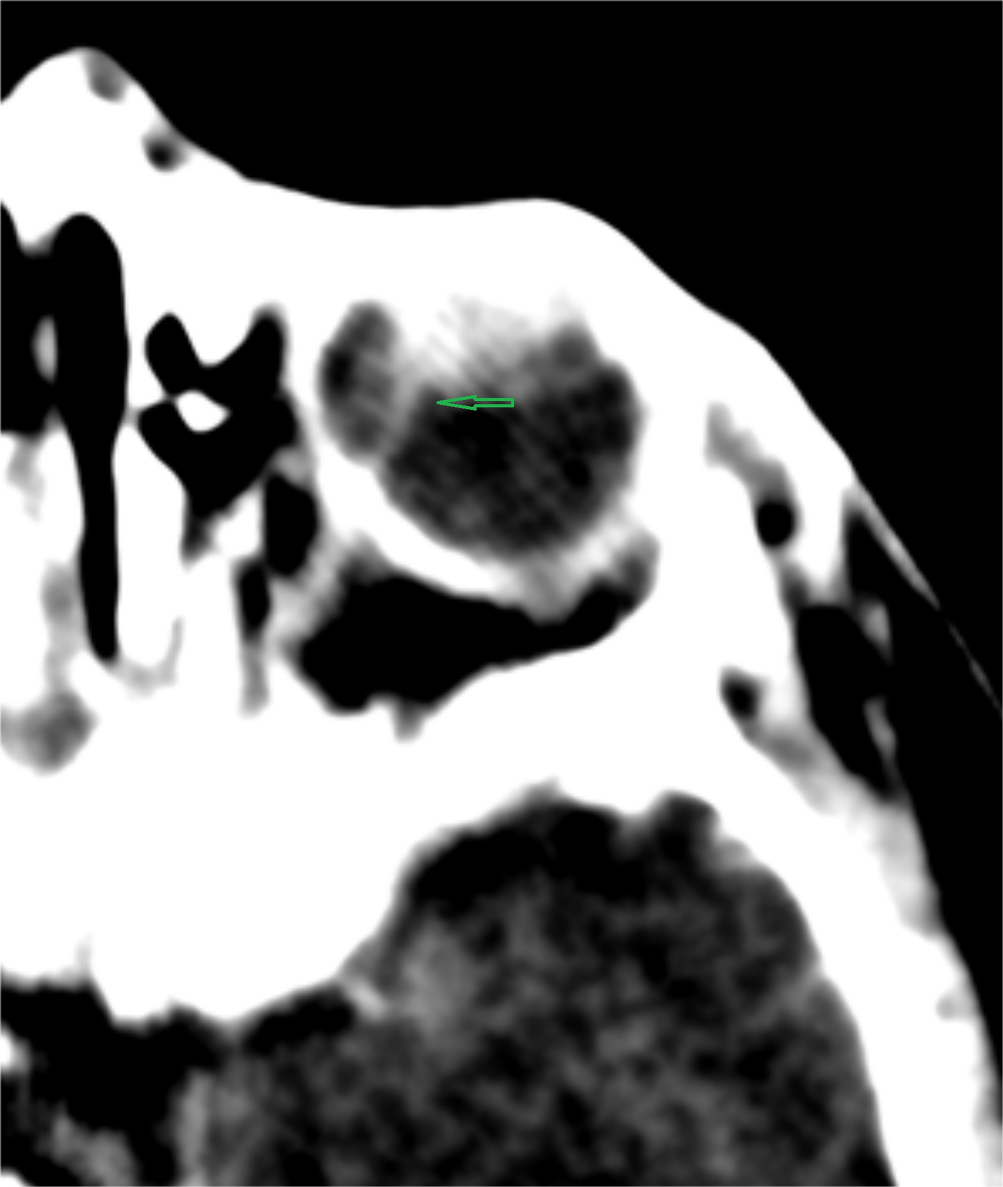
Post-contrast CT scan of the left orbit showing a similar thread-like extension (green arrow) in the posterior segment of the globe CT: computed tomography
